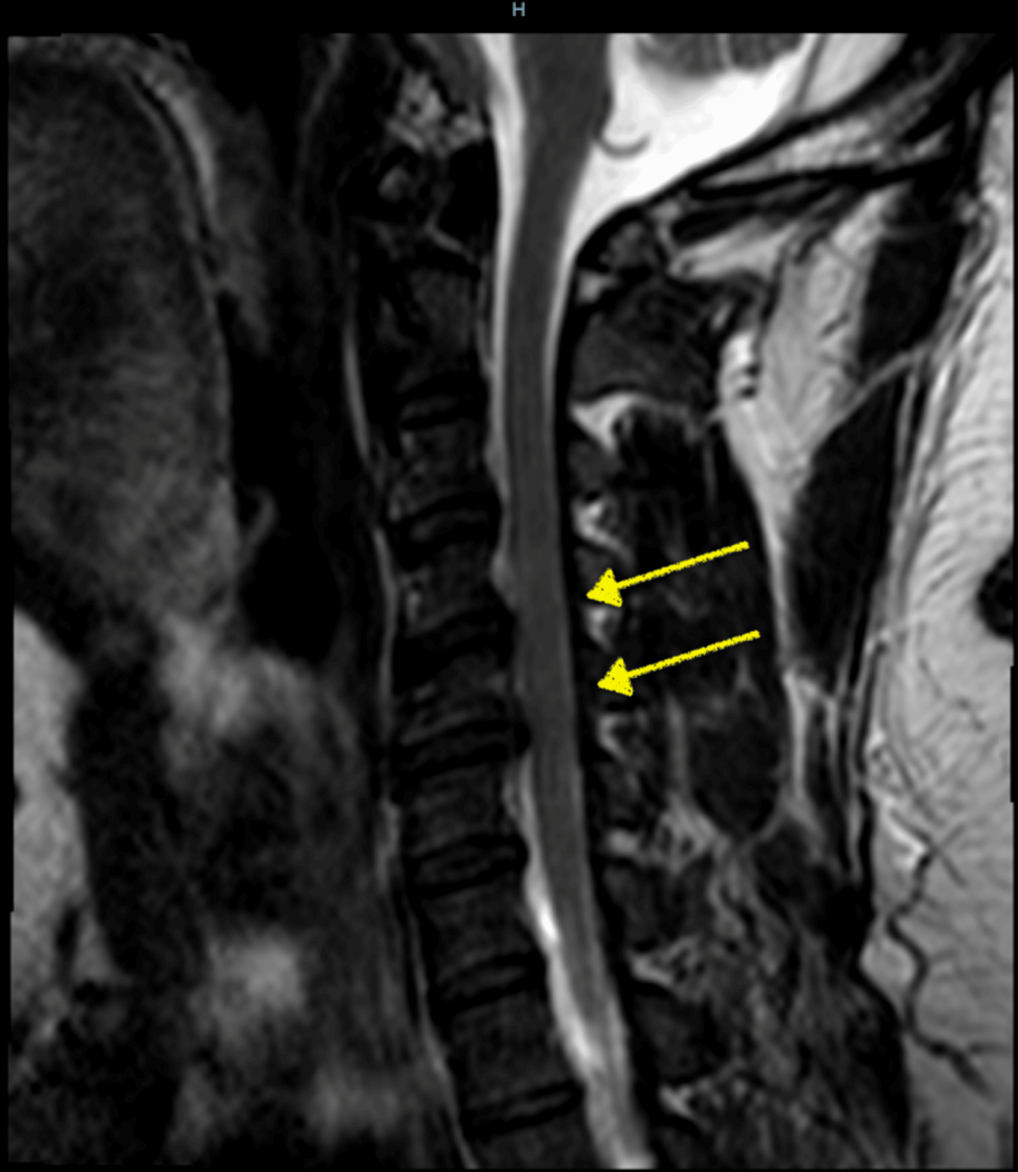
Sagittal view of an MRI of the cervical spine demonstrating stenosis of the spinal canal at C4 through C6 levels (yellow arrows) with absent CSF reserve.
